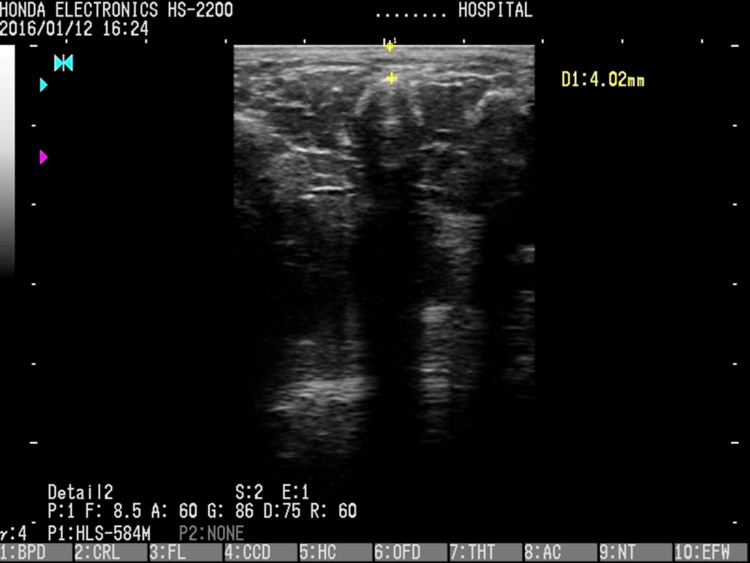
Measure of the thickness of the subcutaneous soft tissue by ultrasound imaging.
